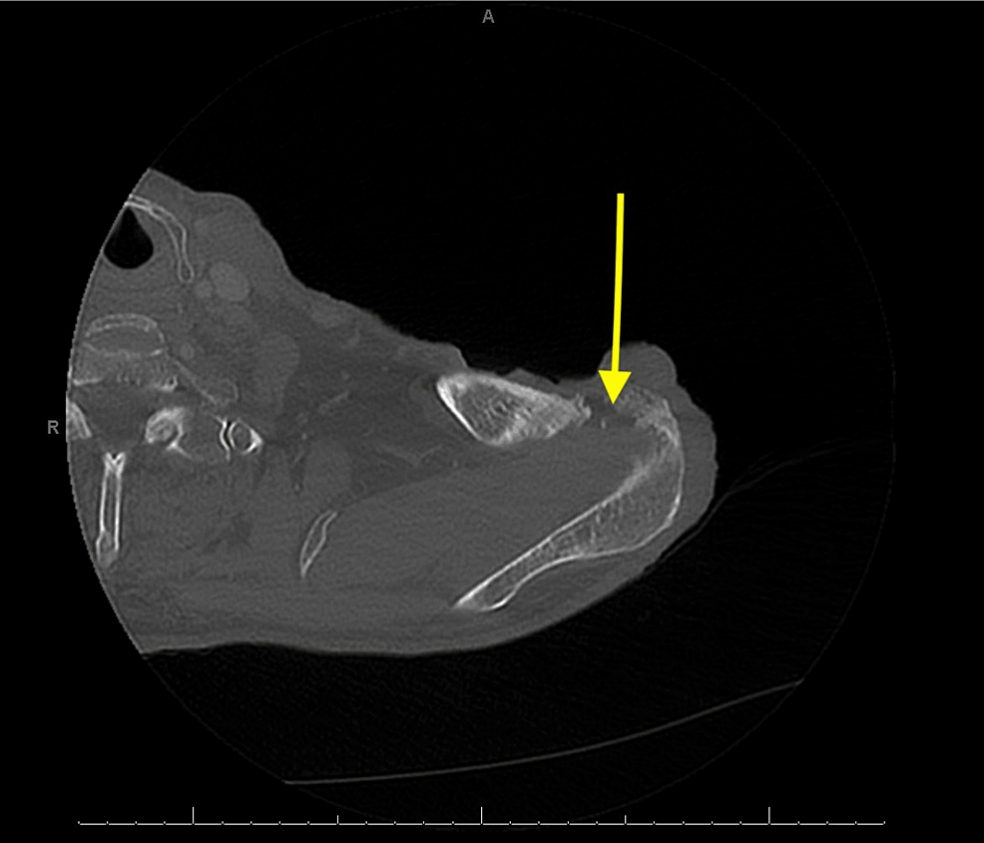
The CT scan of the left shoulder AC joint showing erosive changes (arrow) and soft tissue swelling surrounding the joint. AC: Acromioclavicular
